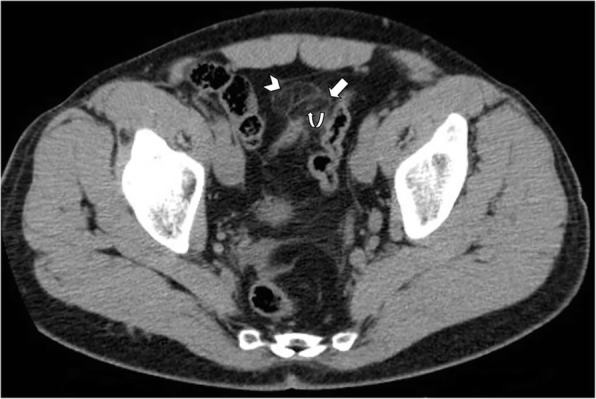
Acute epiploic appendagitis in a 40-year-old man. Axial non-contrast CT image demonstrates a fatty ovoid lesion with high density rim (arrow) that abuts the sigmoid colon and contain central focal area of hyperattenuation (curved arrow), consistent with the “central dot sign” suggestive of thrombosed vascular pedicle. Note also surrounding mild fat inflammation (arrowhead)
